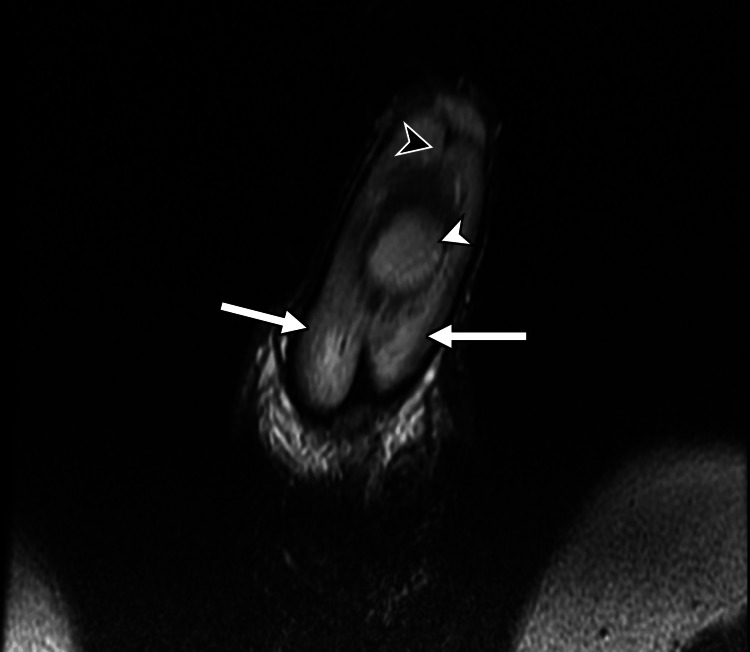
Coronal T2-weighted penile MRI A midline T2-bright collection (white arrowhead) is demonstrated centred over the penile septum (black arrowhead). The penile corpora (white arrows) are surrounding the central septal haematoma.
